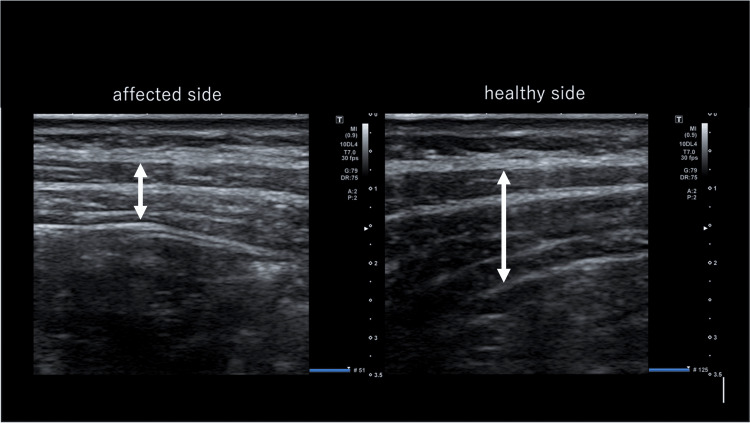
Ultrasonography image. Thinning of the abdominal muscles was observed on the affected side compared with the healthy side. The arrow indicates the muscle layer.
